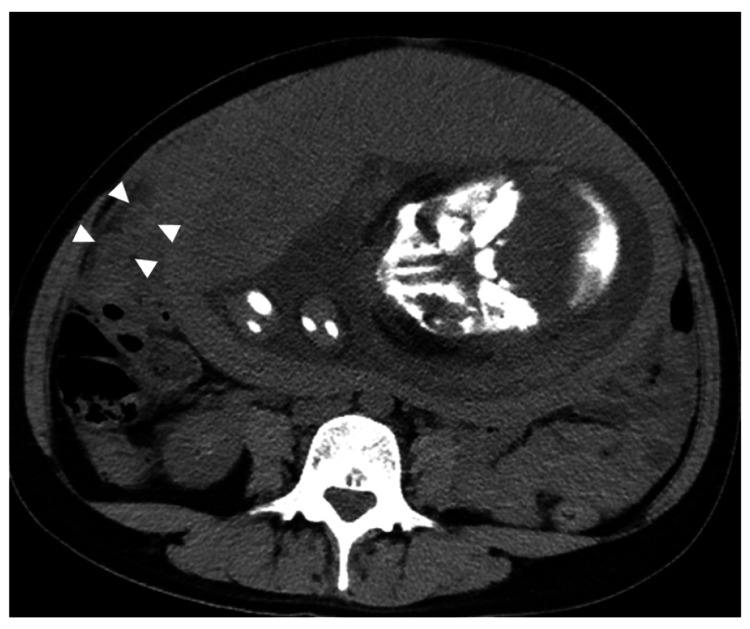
Abdominal computed tomography revealing the enlarged appendix at the right upper quadrant of the abdomen and spread of the inflammation to the surrounding areas (white arrowheads).
